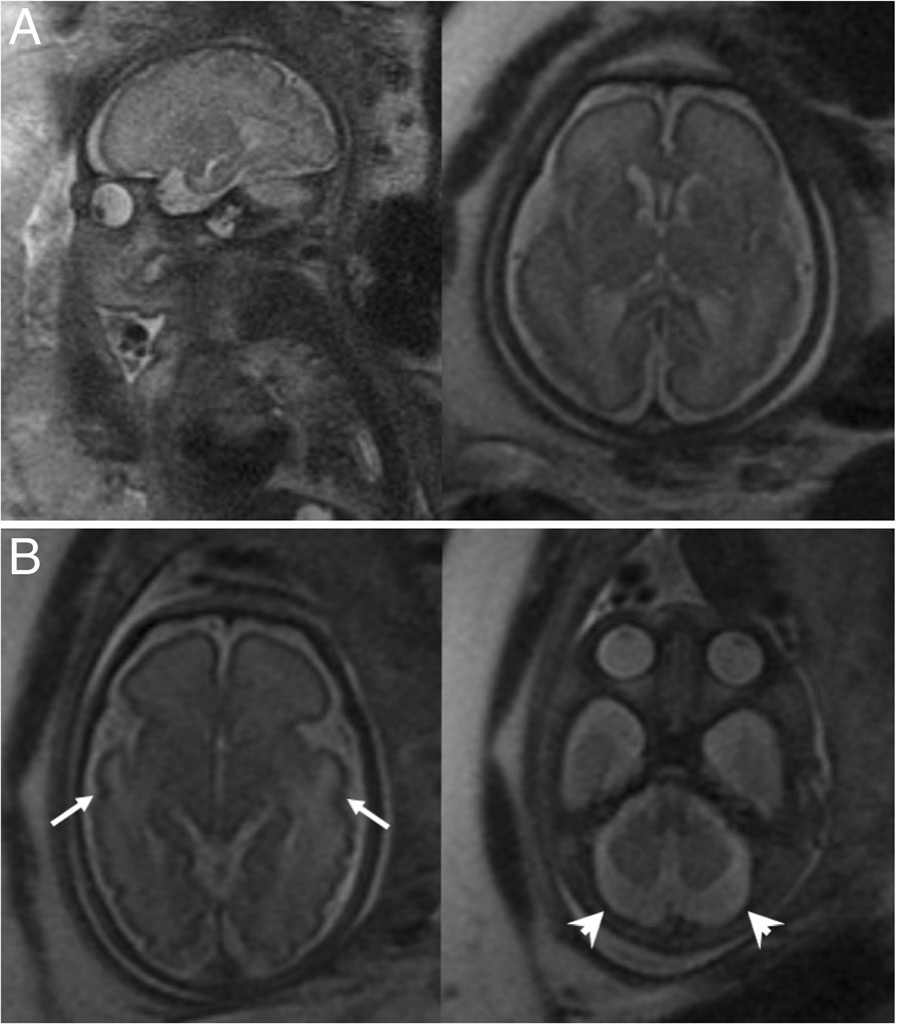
Images of fetal ultrafast MRI. a Patient III-1 at 34 weeks of gestation shows pachygyria, especially in the bilateral frontal lobes, and poor myelination of the white matter (b) Patient III-2 at 32 weeks of gestation shows hypomelination with T2 hyperintensity in bilateral cerebral white matter, particularly both temporal lobes (*arrows*), and prominence of retrocerebellar cisterns due to cerebellar atrophy (*arrowheads*)
